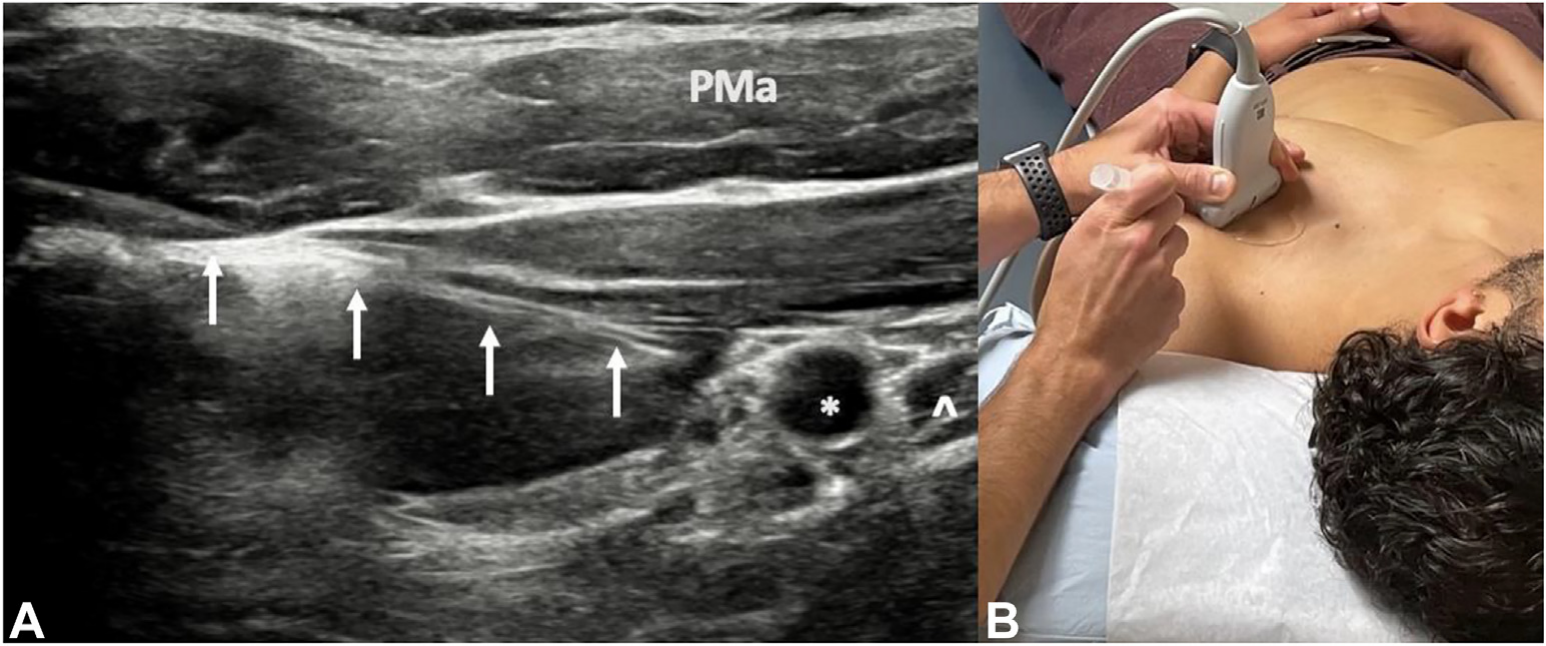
Ultrasound-guided injection of local anesthetic targeted to the PM insertion on the coracoid. The ∗ indicates coracoid, ˆ indicates PM insertion, and white upward arrows indicate needle). PMa, pectoralis major.
